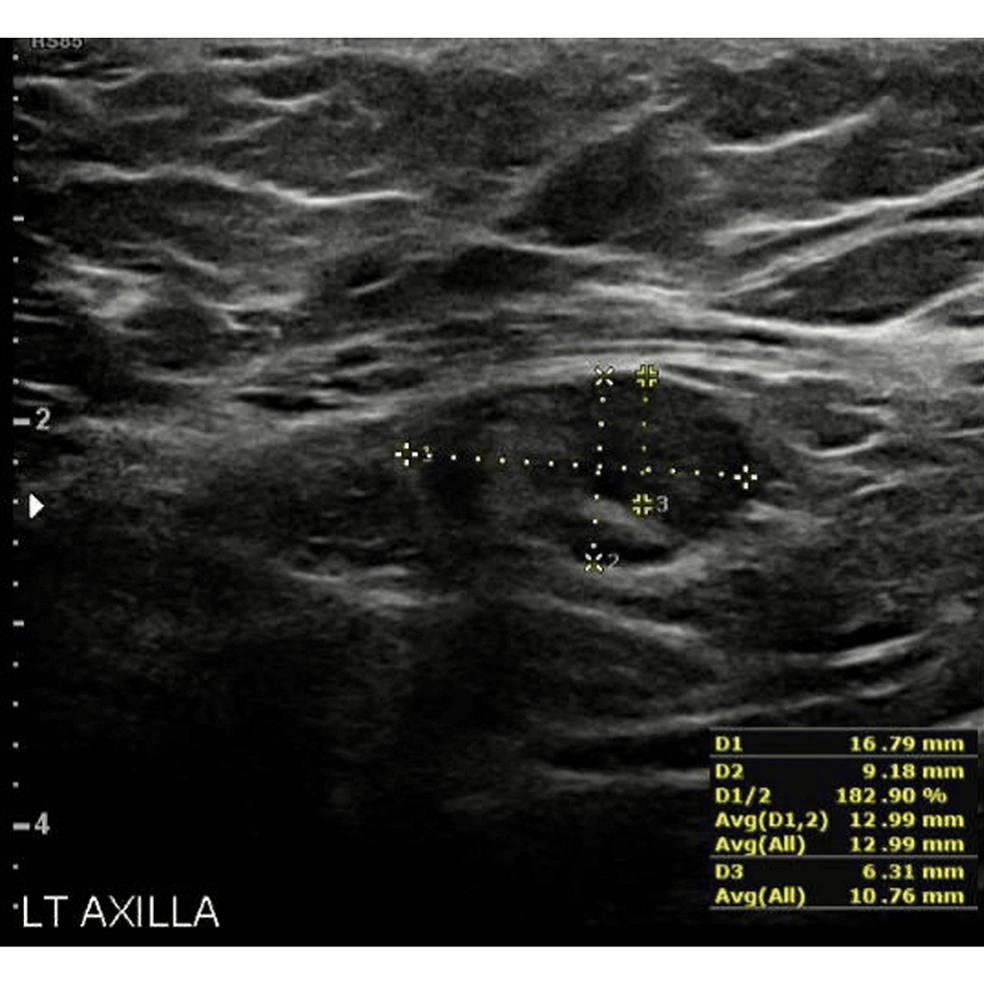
Ultrasound image of a malignant lymph node This figure was obtained from the KFUH system (author's own).
